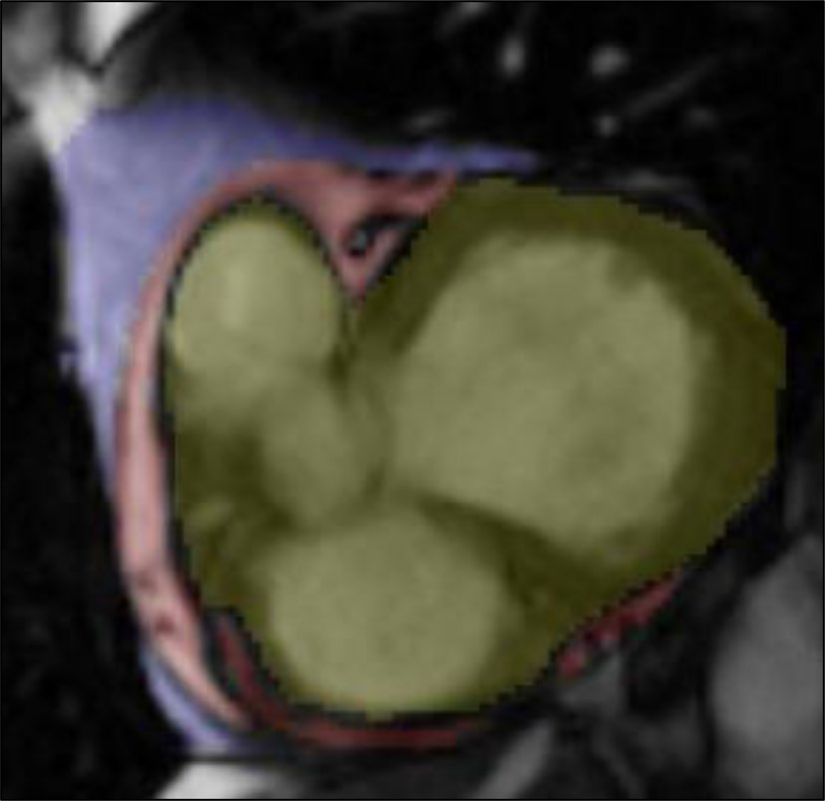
MRI, short-axis slice. Pericardial adipose tissue (blue), epicardial adipose tissue (red), heart volume (yellow)
